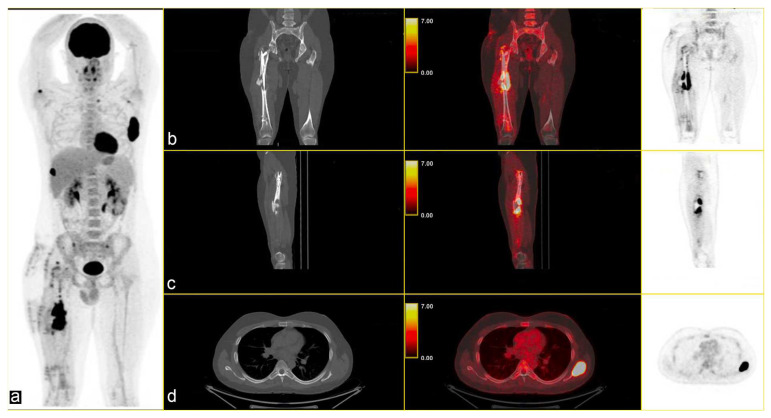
(a) Maximum Intensity Projection (MIP) shows several skeletal lesions at different sites; CT-PET fusion images: (b,c) sagittal images of the primary lesion in the left femur and (d) an osteolytic lesion (SUV_max_ 35) in the left scapula (b–d): CT on the left, CT fused images in the middle, and PET image on the right.
